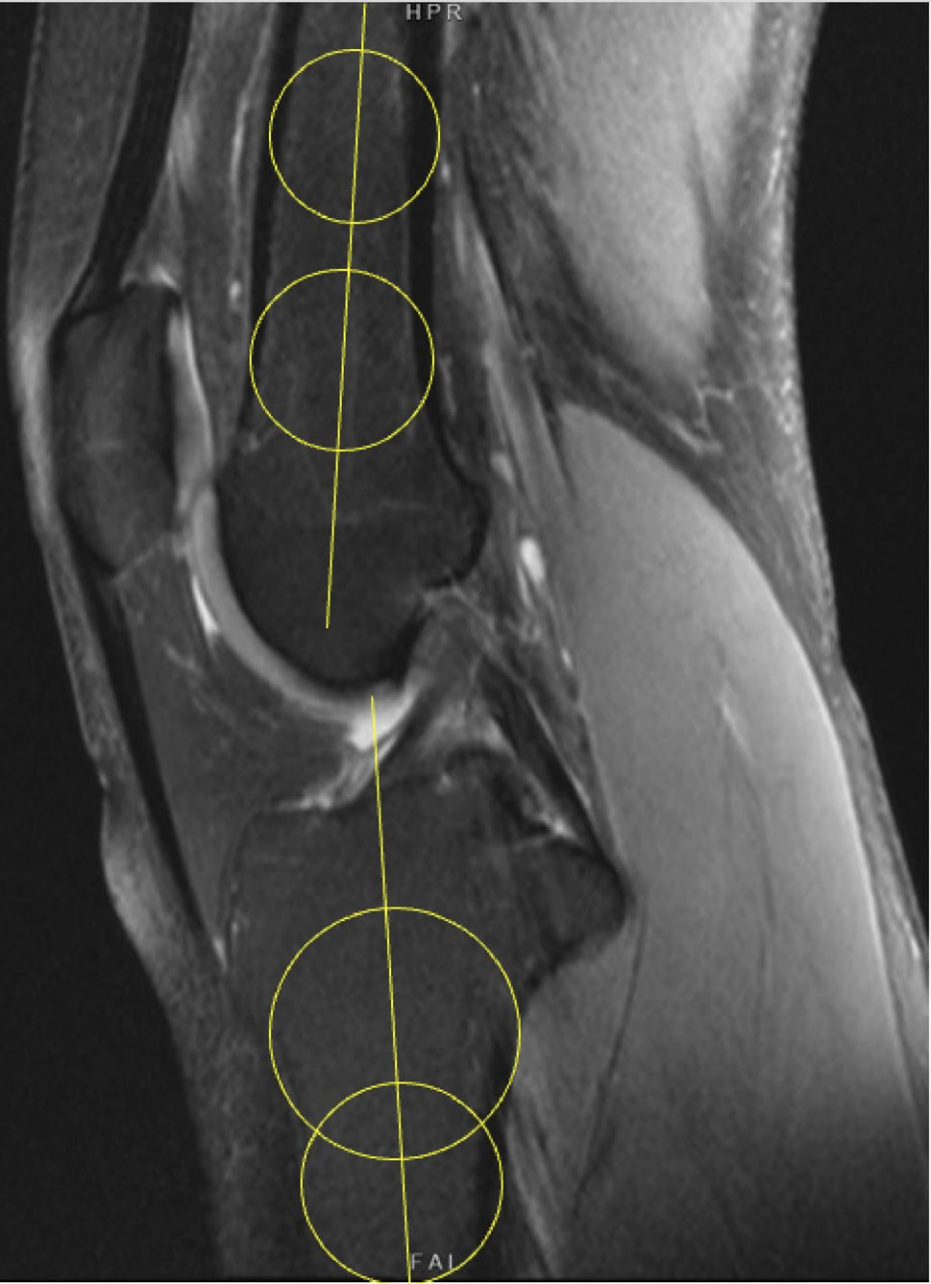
MRI-based measurement of the knee flexion angle
